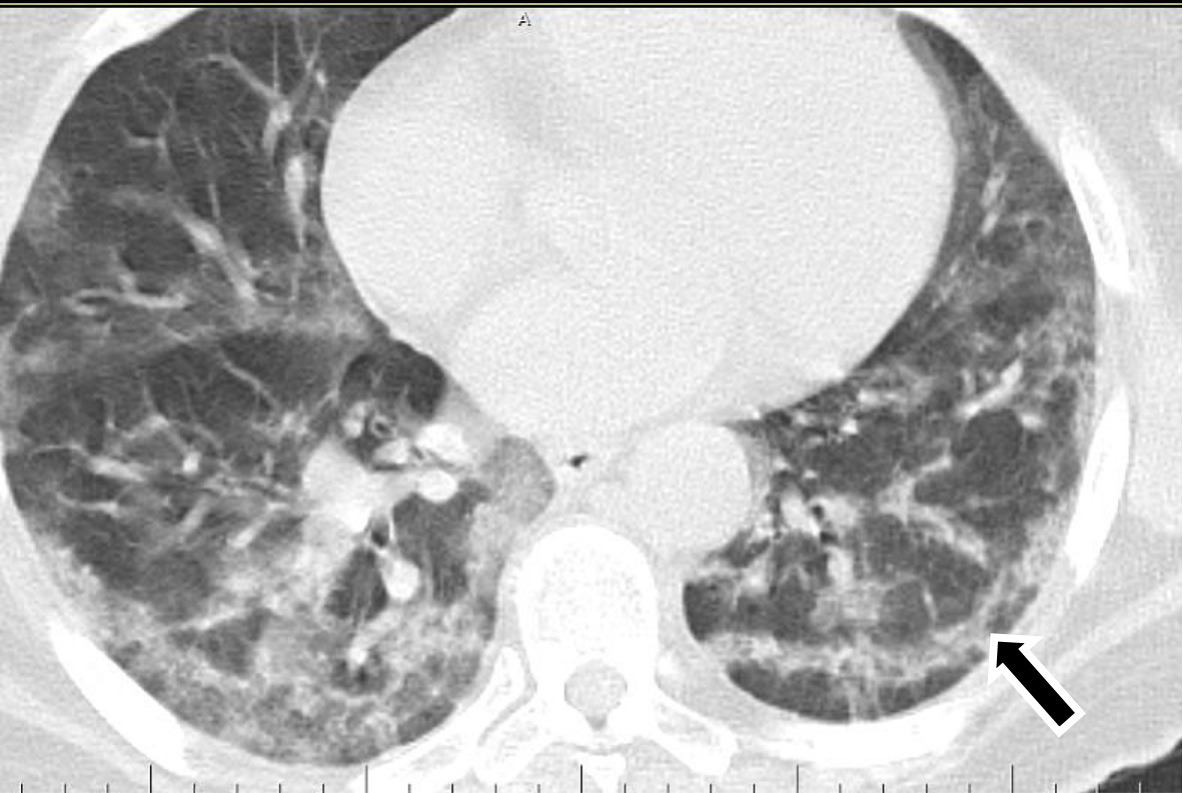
A 60-year-old female patient with COVID-19. CT chest axial image shows bilateral subpleural crazy paving, architectural distortion, and perilobular thickening. A subpleural band is seen on the left (black arrow)
